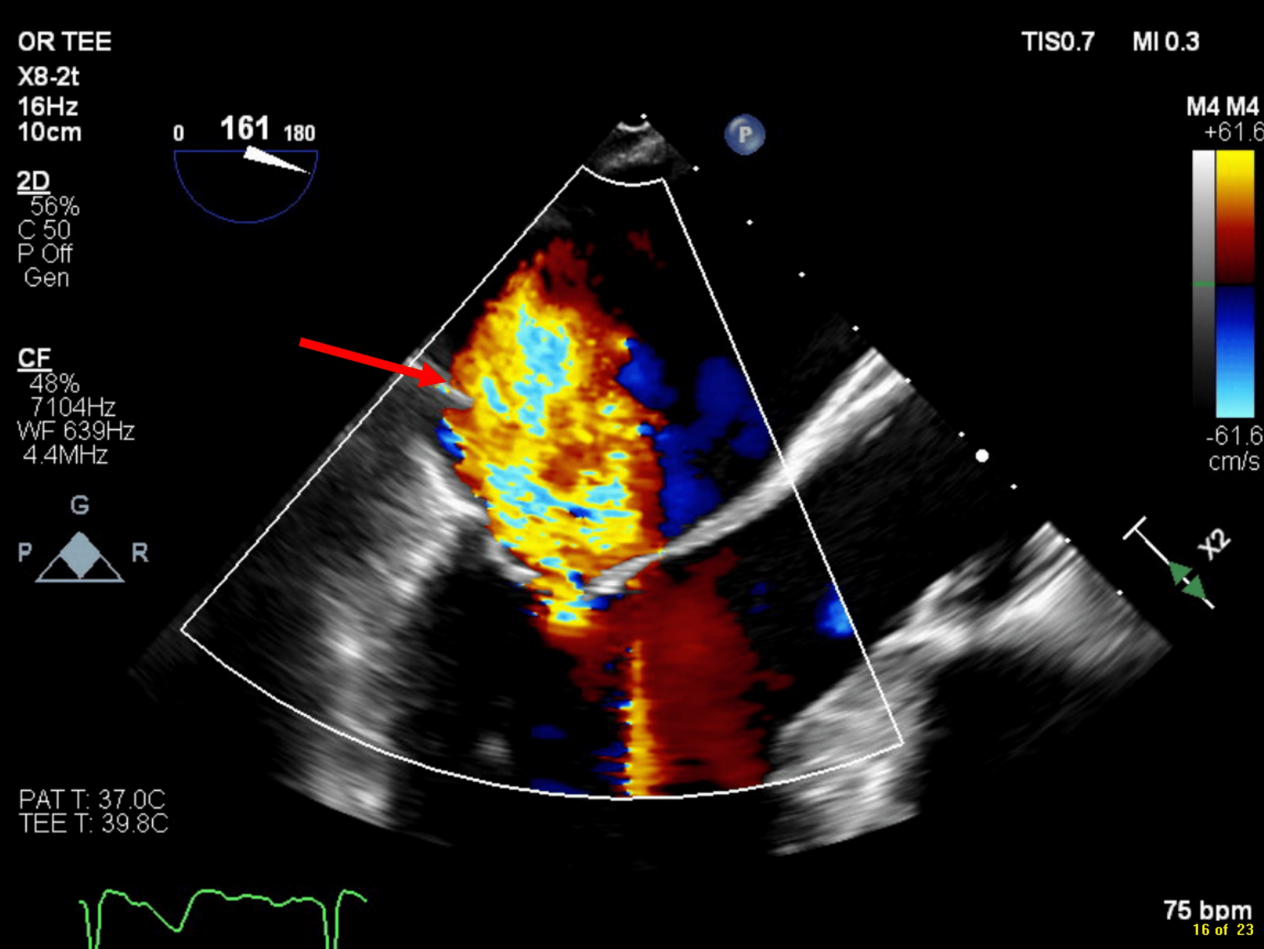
Central component of mitral regurgitation jet. Midesophageal long-axis view obtained with transesophageal echocardiography showing the central component of the mitral regurgitation jet (arrow).
